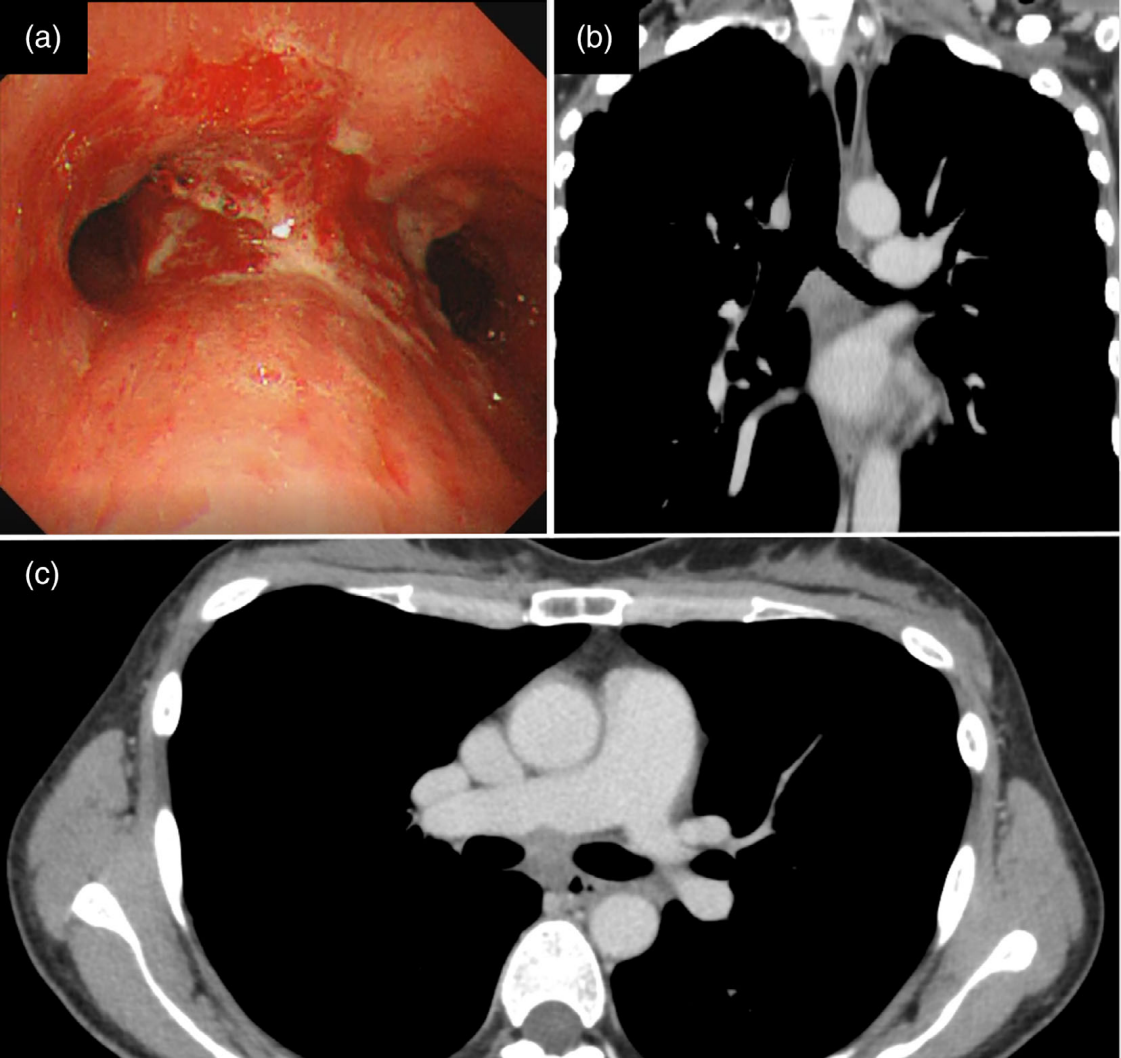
(a) Endoscopic examination after three months of alectinib therapy showing opening of the left main bronchus; and (b,c) computed tomography six months after alectinib therapy demonstrating marked reduction of the tumor.
